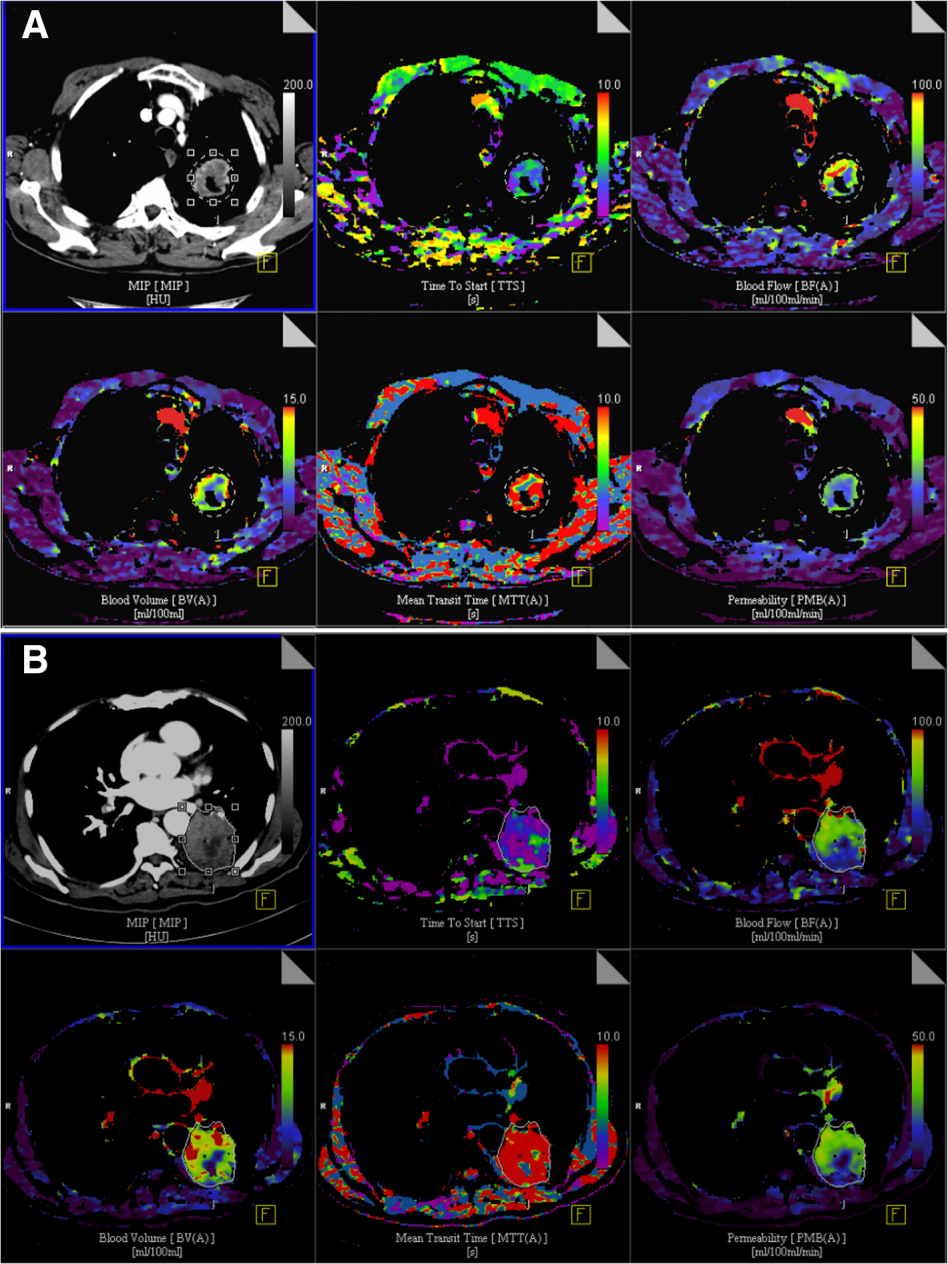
The dVPCT indirectly demonstrated thick-wall cavitary ADC were more hypoxic than non-cavitary ADC. The tumor permeability, blood flow, blood volume and mean transit time was higher in patients with a non-cavitary ADC than b thick-wall cavitary ADC. Abbreviations: dVPCT, the dynamic volume perfusion CT
